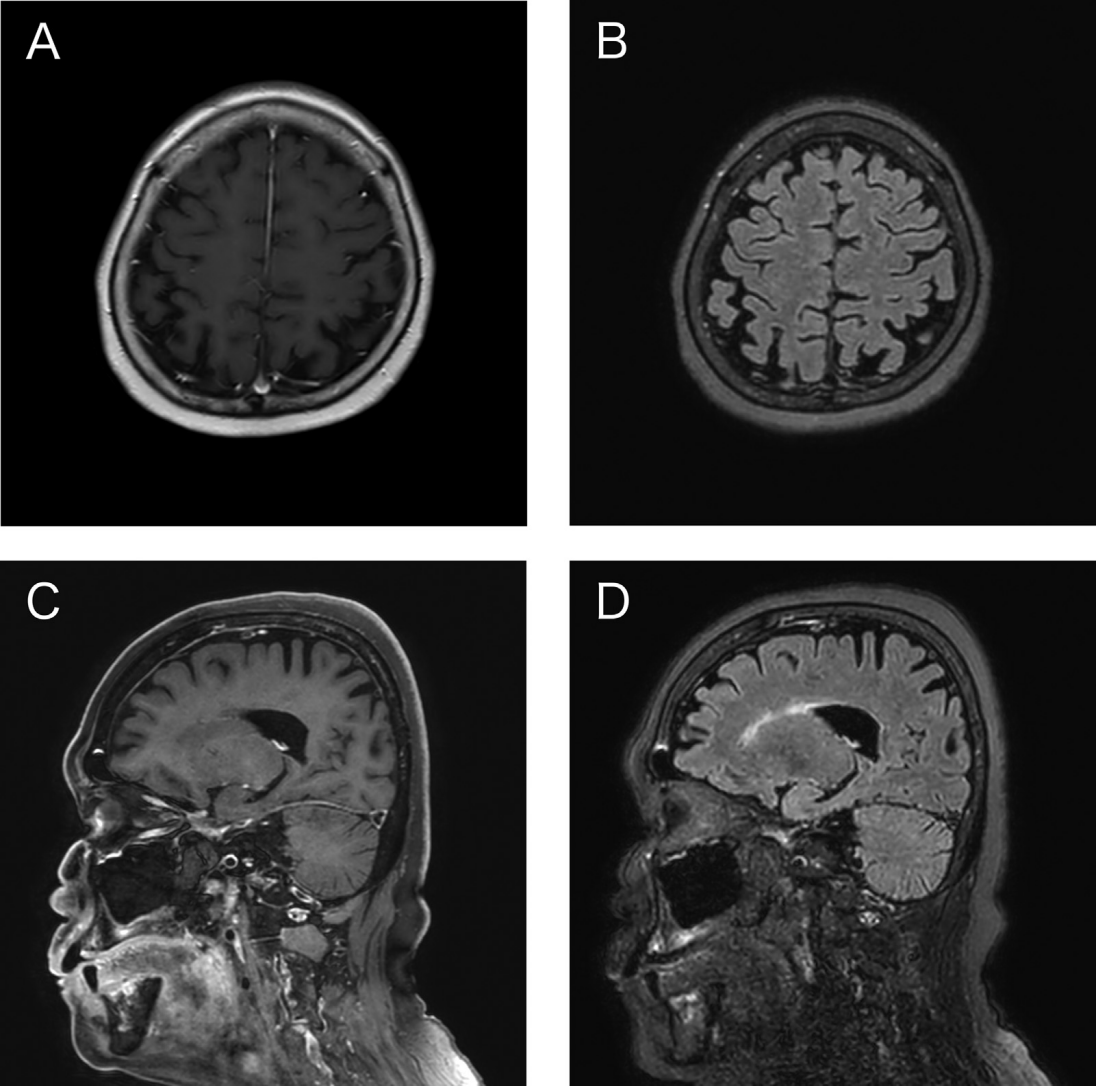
(A, C) CE-T1WI (B, D) CE-FLAIR CE-MRI performed 6 months post-treatment showed marked improvement in the thickened dural enhancement. While CE-T1WI demonstrated normal dural enhancement, CE-FLAIR revealed complete absence of enhanced dural matter.
